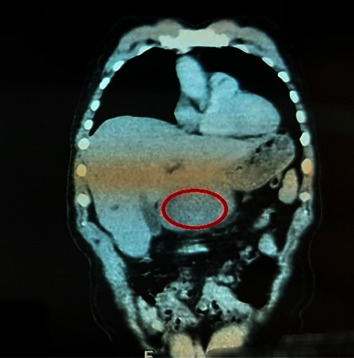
Reformatted coronal contrast-enhanced CT demonstrates a large cystic lesion with a relatively thin wall in the hepatic segment IVb. CT findings are compatible with hepatic abscess.
